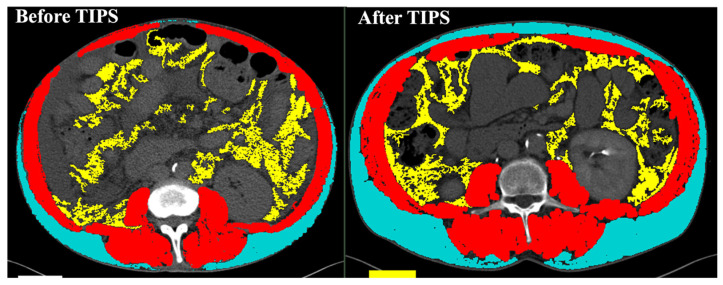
Modifications of muscle mass and adipose tissue after TIPS placement. Contrast-enhanced abdominal CT scan of a 72 year old male cirrhotic patient submitted to TIPS for treatment of refractory ascites. Six months after TIPS placement, the skeletal muscle area (red) increased from 15,976 cm^2^ to 19,266 cm^2^ with an increase in SMI from 49.3 cm^2^/m^2^ to 59.4 cm^2^/m^2^; parallelly, subcutaneous adipose tissue (SAT) (light blue) increased from 5649 cm^2^ to 13,248 cm^2^ with an increase in SATI from 17.4 cm^2^/m^2^ to 40 cm^2^/m^2^. Visceral adipose tissue (VAT) (yellow) decreased from 9202 cm^2^ to 7809 cm^2^ with a decrease in VATI from 28 cm^2^/m^2^ to 24 cm^2^/m^2^.
